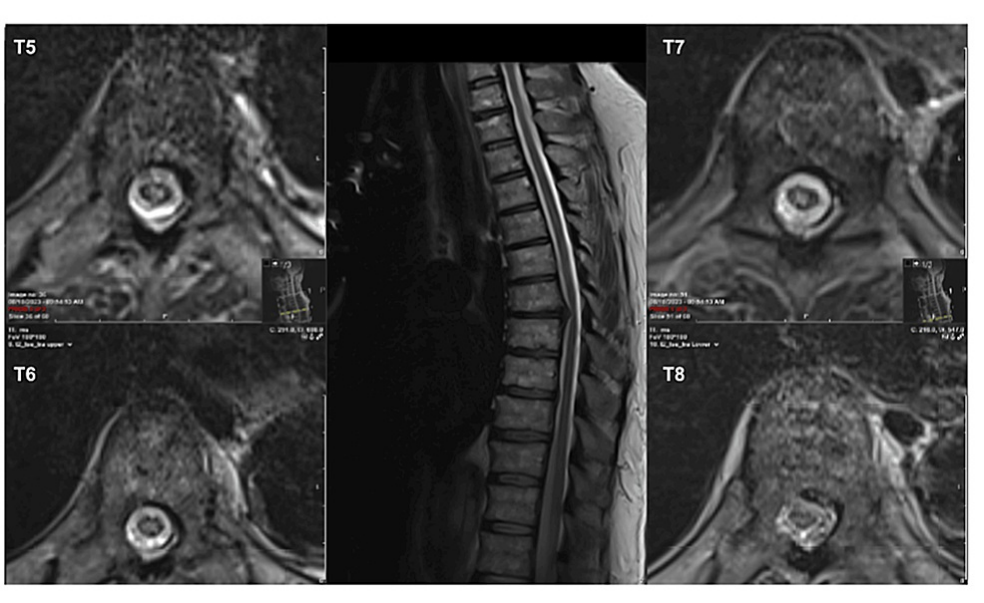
T2 sequences of an MRI thoracic spine Sagittal (center) and axial cuts through the mid-vertebral body at various levels (labeled) on post-injury day four demonstrating T2 signal change throughout the spinal cord, suggestive of an ischemic event.
